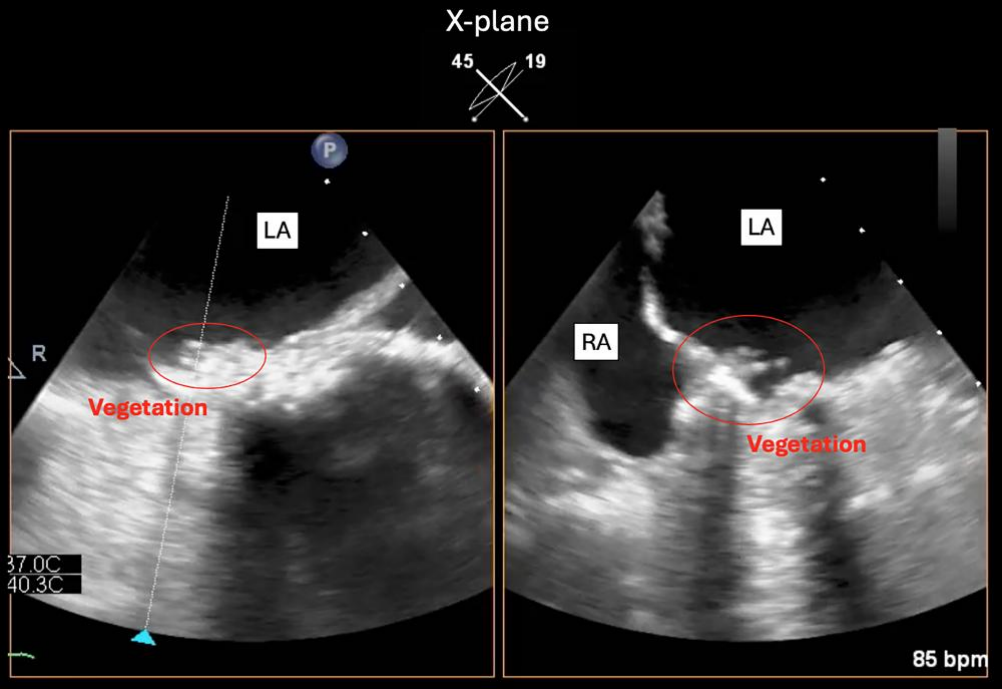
Transoesophageal echocardiogram (*x*-plane mode with 45° and 19° angles) showing vegetations on the right atrioventricular mechanical valve (in the circle). LA, left atrium; RA, right atrium.
